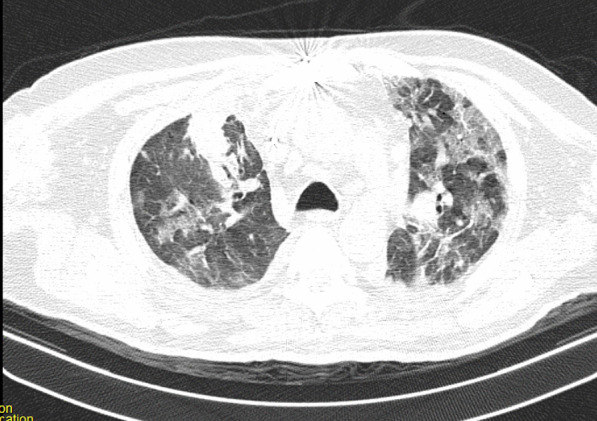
Chest CT recommended considering infection with COVID-19
